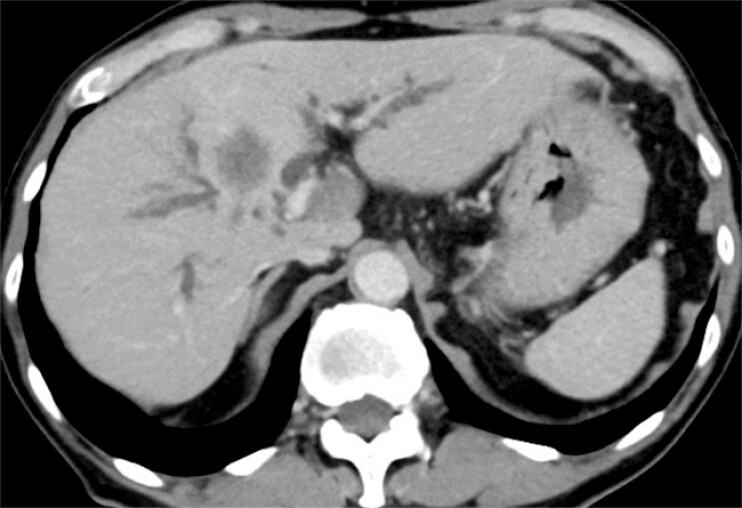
Computed tomography showed a perihilar biliary stricture due to the intrahepatic cholangiocarcinoma.
